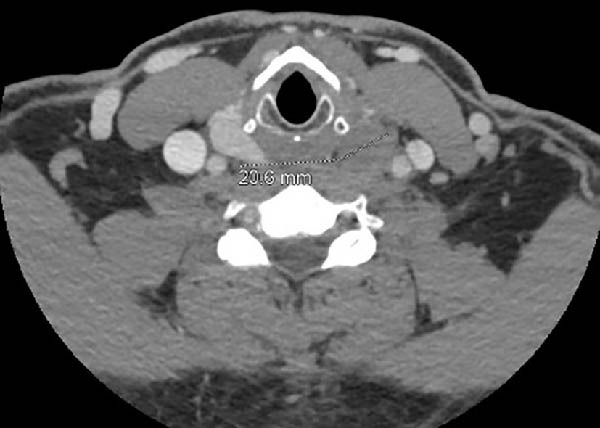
CT of the neck showing mass‐like fullness in the left compartment of the neck.
